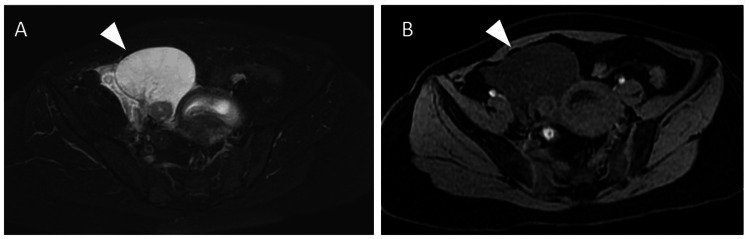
Pelvic magnetic resonance imaging findings A: Axial T2-weighted image. A high signal is observed in the stromal region of the right ovary. B: Axial T1-weighted image. No sign of bleeding or hematoma in the right ovary is evident.
